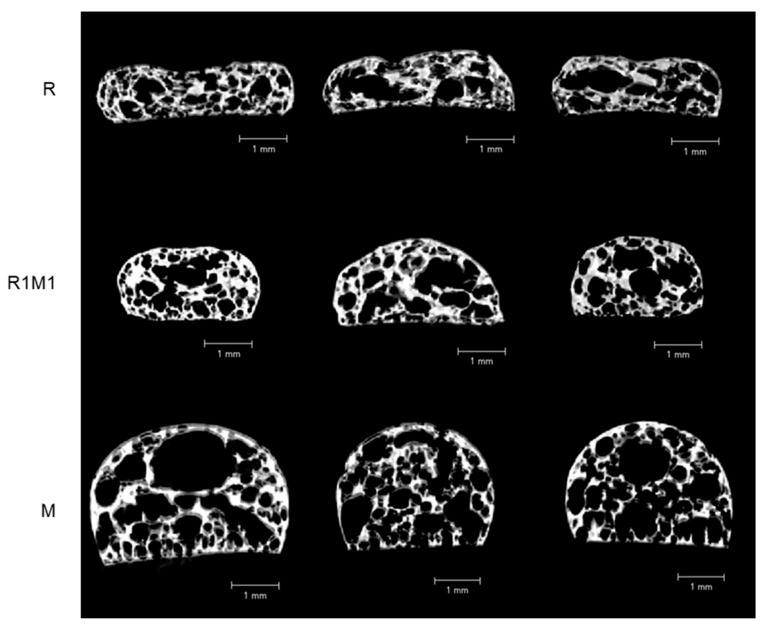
Cross-sectional 2D X-ray micro tomography images of printed single-layer samples after baking. The images represent the area of the sample where the hardness measurement by cutting was performed.
